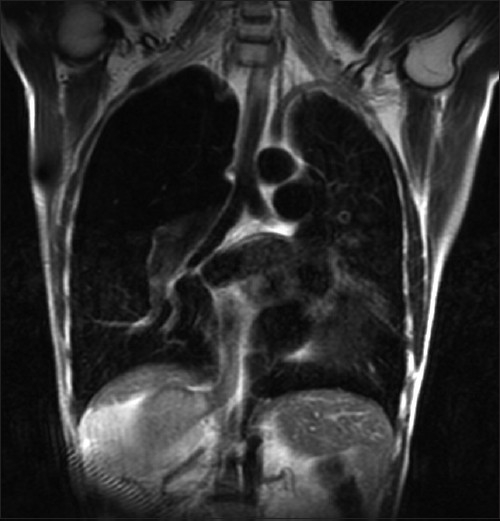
Coronal T2-weighted MRI shows bilateral hyparterial bronchi with emphysematous right upper lobe.
